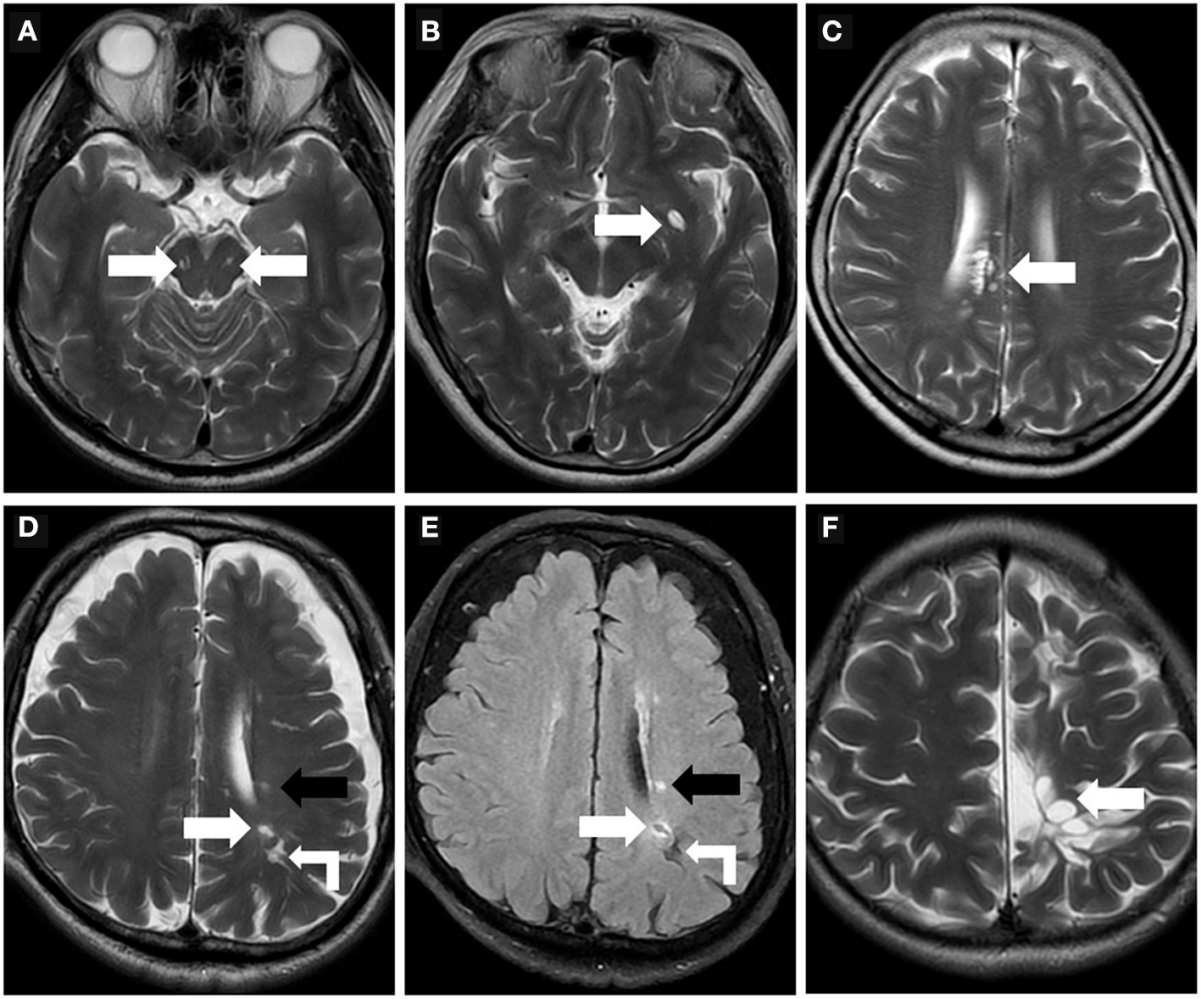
Morphologic characteristics of the perivascular spaces. (A) Type III-midbrain type: Axial T2WI showed multiple linear EPVS (white arrow) with high intensity in the midbrain, which were generally symmetrically distributed on both sides and consistent with the course of the penetrating arteries. (B) In a 62-year-old woman, axial T2WI showed a large EPVS in the left precribrum with sac-like high intensity, within which a linear hypointense vessel can be seen, namely “vessel sign” (white arrow). (C) Axial T2WI revealed multiple linear and ovoid EPVS clustered locally near the right lateral ventricle (white arrow). (D,E) In a 76-year-old man with a history of hypertension and diabetes mellitus for many years, EPVS (white right angle arrow), WMH (black arrow), and small lacune (white arrow) were simultaneously present in the left centrum semiovale. The lacune (white arrow) showed a hyperintense rim around the vesicular cavity on FLAIR sequence, which can be distinguished from EPVS. (F) A 32-year-old male with cerebral cleft malformation combined with hypoplasia of the corpus callosum in the left cerebral hemisphere, who had recurrent headaches for many years. Multiple tumefactive PVS (white arrow) were seen in the subcortical white matter areas, and the lesions were unchanged on follow-up reviews of brain MR.
